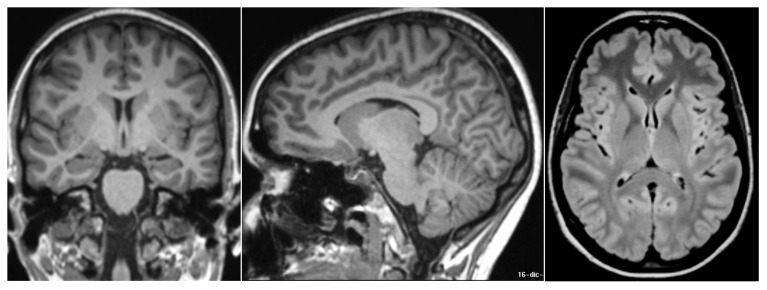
Normal findings at brain magnetic resonance imaging (MRI). Coronal and sagittal T1-weighted and axial FLAIR images show absence of mesial temporal lobe and cortical of atrophy and absence of signal changes.
